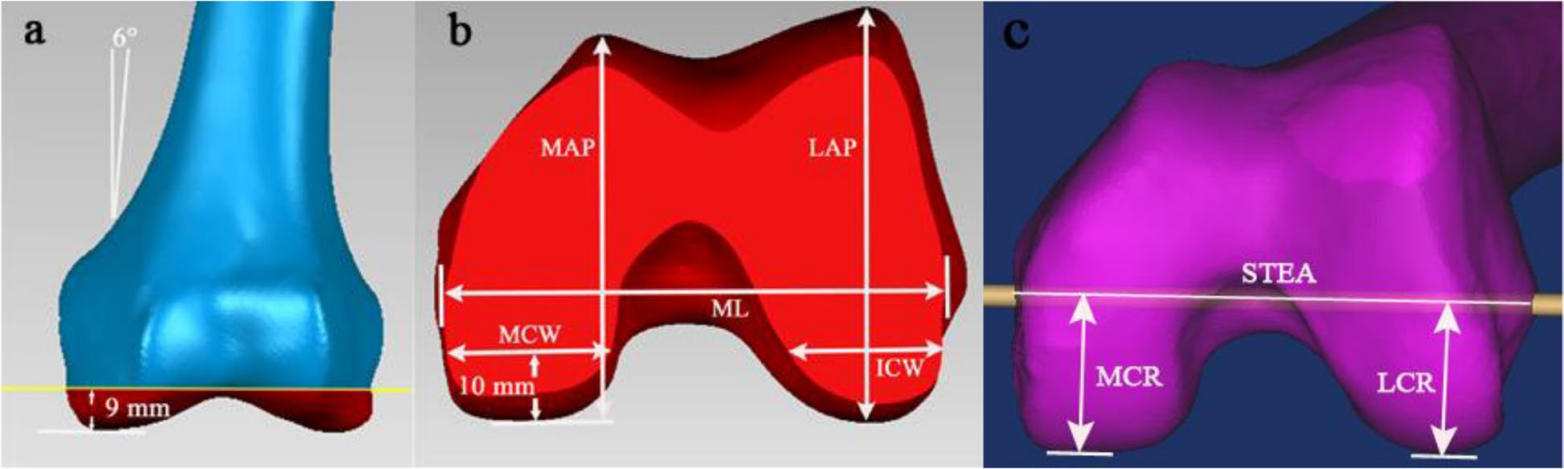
Distal femur resection and measurement on CT images. a Resection method of distal femur. b Measurements of resected femoral surfaces. c Measurements of femoral posterior condyle curvature radii
